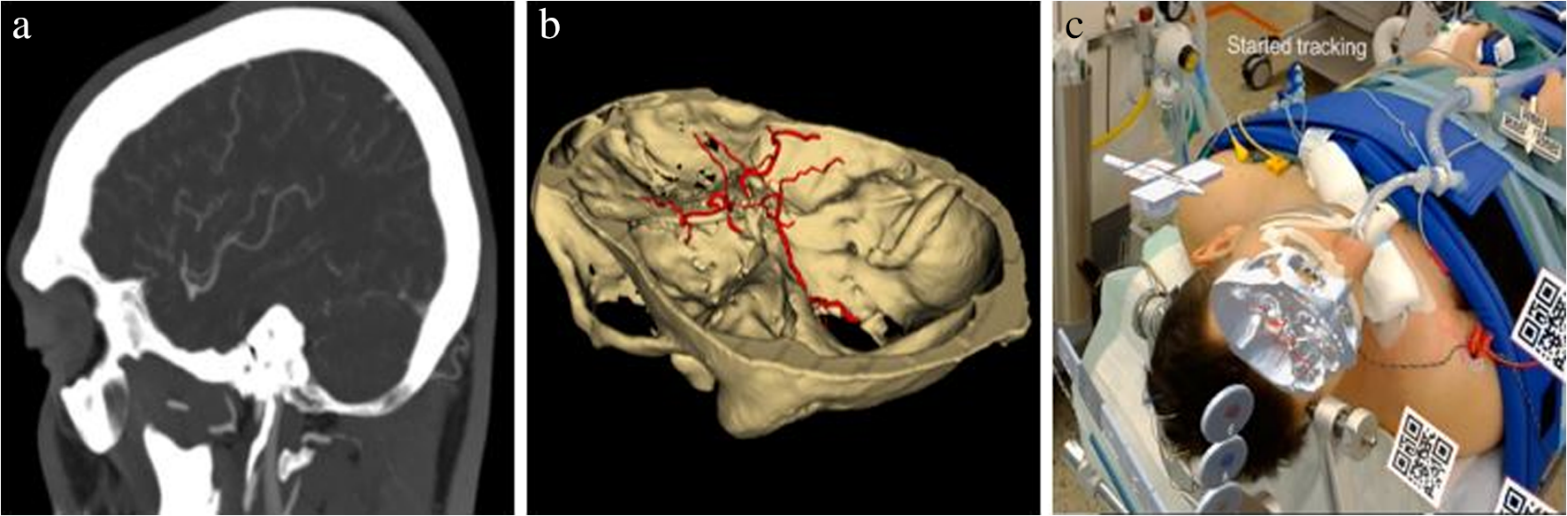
(a) CT-image of MCA aneurysm, sagittal view (b) Holograms of skull base and circle of Willis including MCA aneurysm on the left side (c) Holograms after registration as seen through the HoloLens
